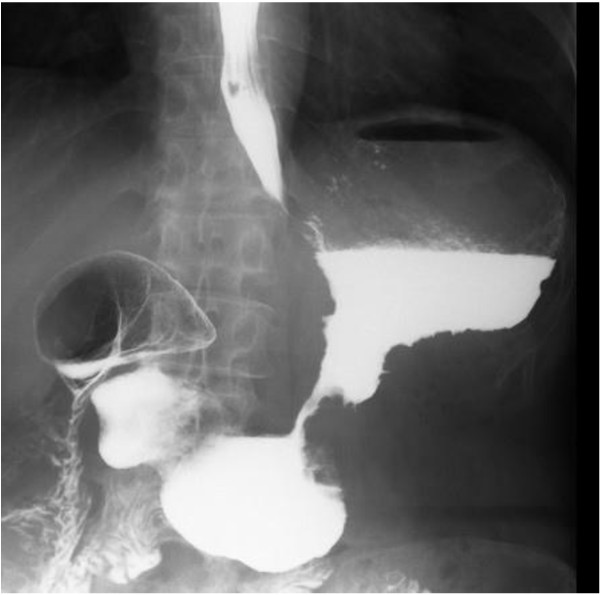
Contrast radiography of our patient on their initial visit. Scirrhous type gastric cancer was distributed in the wide area of the stomach with stricture of the gastric body seen on contrast radiography.
